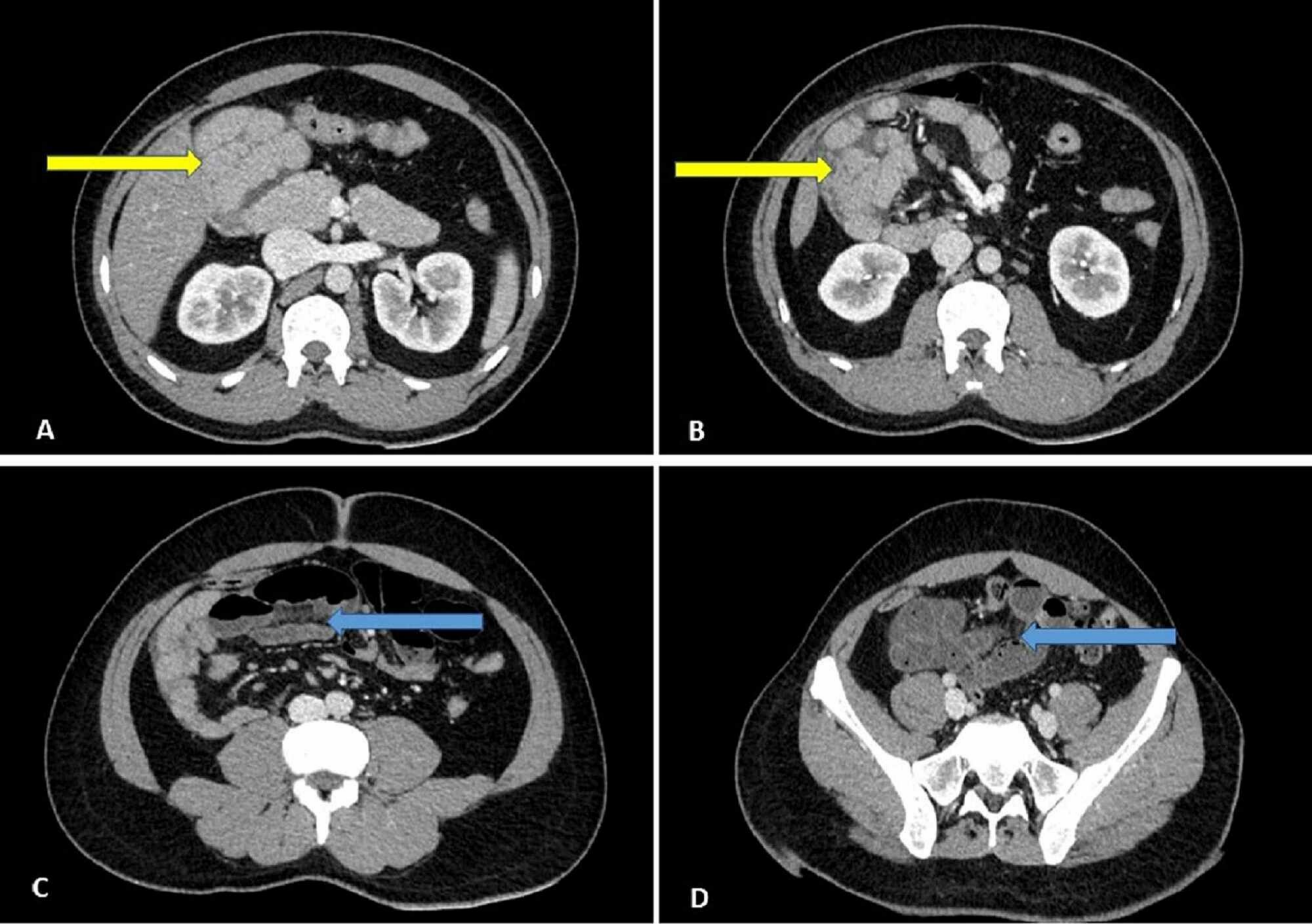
Coronal sections of the contrast-enhanced CT scan of the abdomen demonstrating a cluster of small bowel loops in the right side of the abdomen (yellow arrows). The ischemic bowel loops (blue arrows). CT scan - Computed Tomography scan
